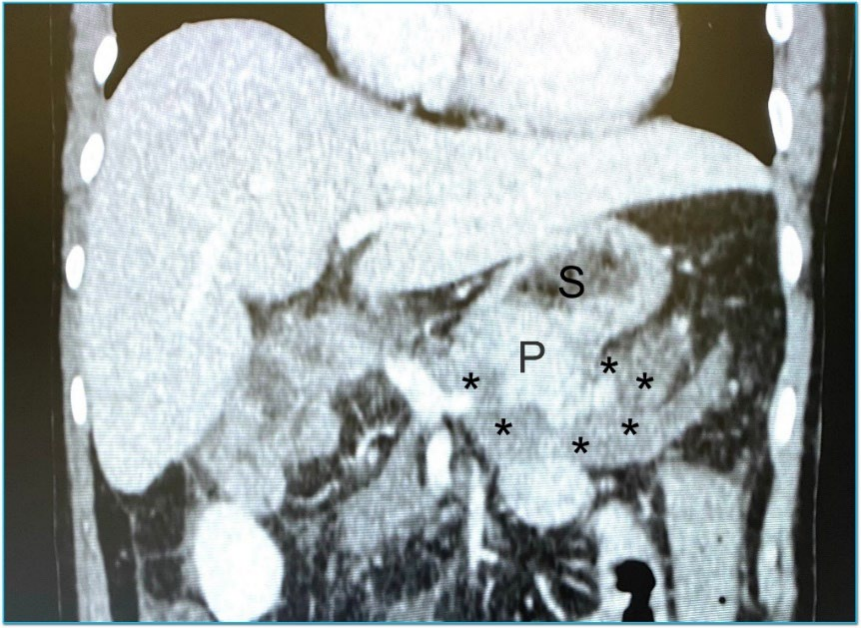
CT scan of a patient with nausea, vomiting, and leukocytosis on postoperative day 7. (S) stomach; (P) pancreas remnant. *Peripancreatic fluid.
